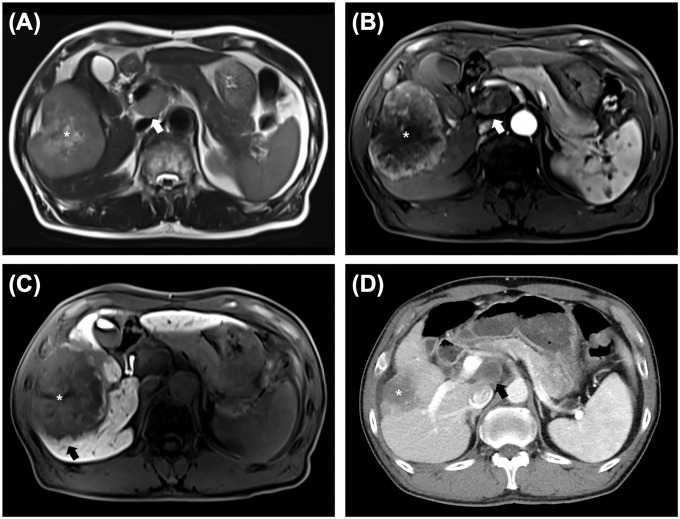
Images in a 62-year-old man with advanced hepatocellular carcinoma. (a) On T2-weighted magnetic resonance (MR) imaging, an 8.3 cm sized mass (asterisk) was seen in the right hepatic lobe. A metastatic lymph node is present in the portal space (white arrow). The mass was confirmed to be an Edmondson grade III hepatocellular carcinoma by biopsy. (b) The arterial-phase MR image shows an absence of peritumoral arterial-phase hyperenhancement around the index tumor (asterisk). Metastatic lymph nodes also show irregular enhancement (white arrow). Portal vein tumor thrombosis was not observed (not shown). (c) In the hepatobiliary phase, peritumoral hypointensity (black arrow) is observed around the index tumor (asterisk). (d) Computed tomography obtained during the portal phase 16.7 months after the atezolizumab-bevacizumab treatment without concomitant radiation therapy shows a shrunken HCC in the right hepatic lobe (asterisk) without other new metastatic lesions.
